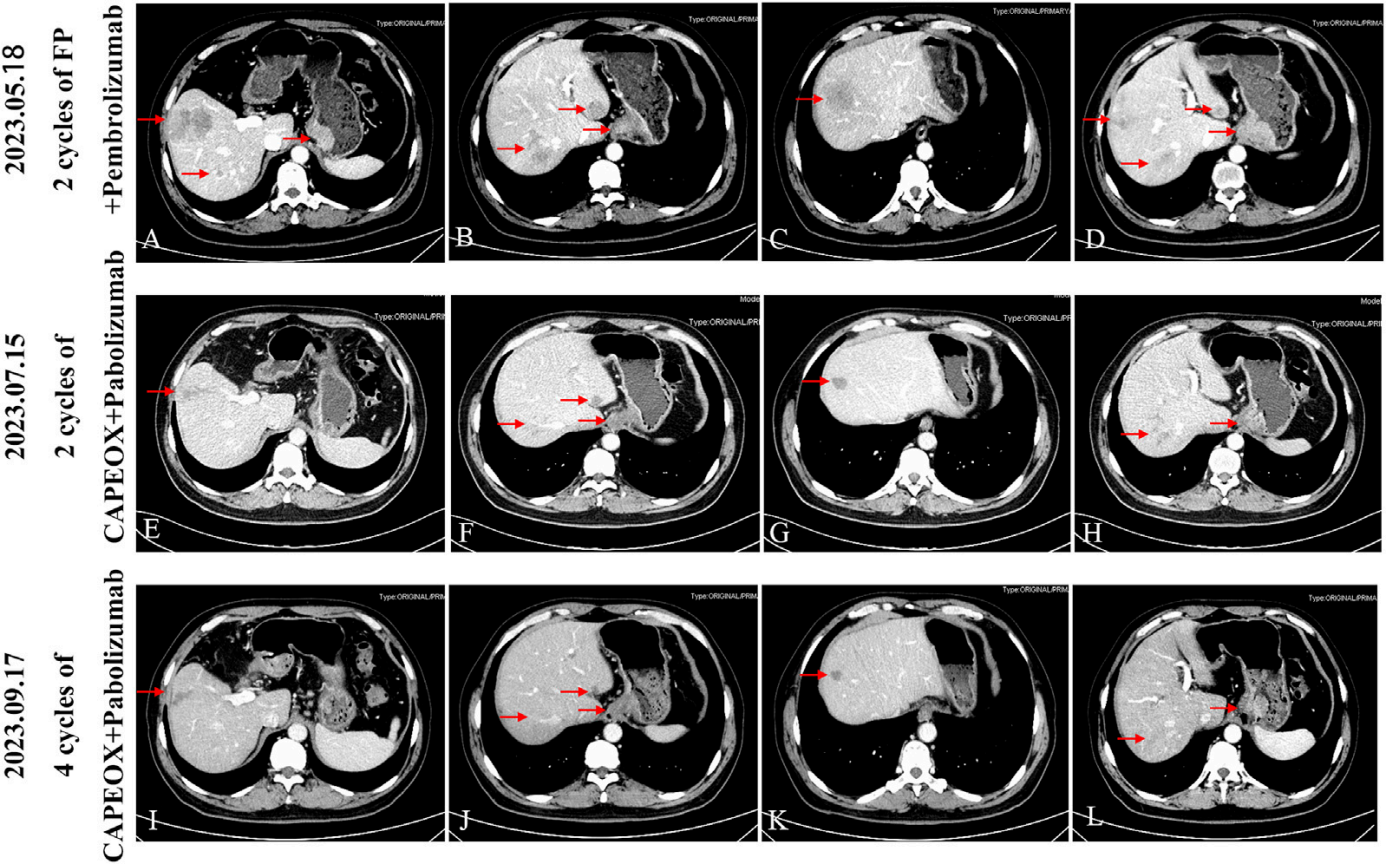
After four cycles of follow-up with CAPEOX combined with pembrolizumab, the efficacy evaluation was sustained partial remission (PR), according to RECIST 1.1. (A-D) Progress (PD) after three cycles of FP regimen combined with parolizumab, follow-up CT scan upon admission to our hospital. (E-H) After receiving CAPEOX combined with pembrolizumab treatment for two cycles in our hospital, the efficacy evaluation was partial remission (PR). (I-L) Four cycles CAPEOX combined with pembrolizumab treatment, the efficacy evaluation was sustained partial remission (PR).
